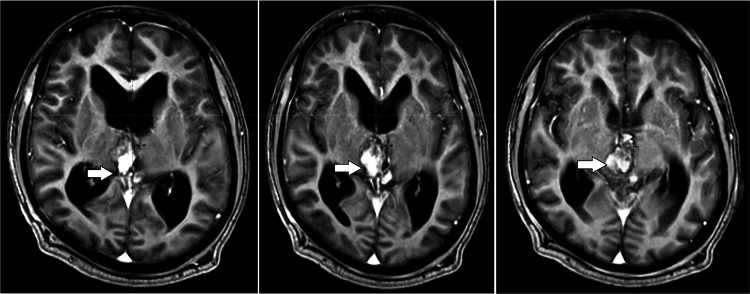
Postoperative T1 contrast MRI axial images showing the residual tumor in the pineal region after the subtotal resection of the tumor.
